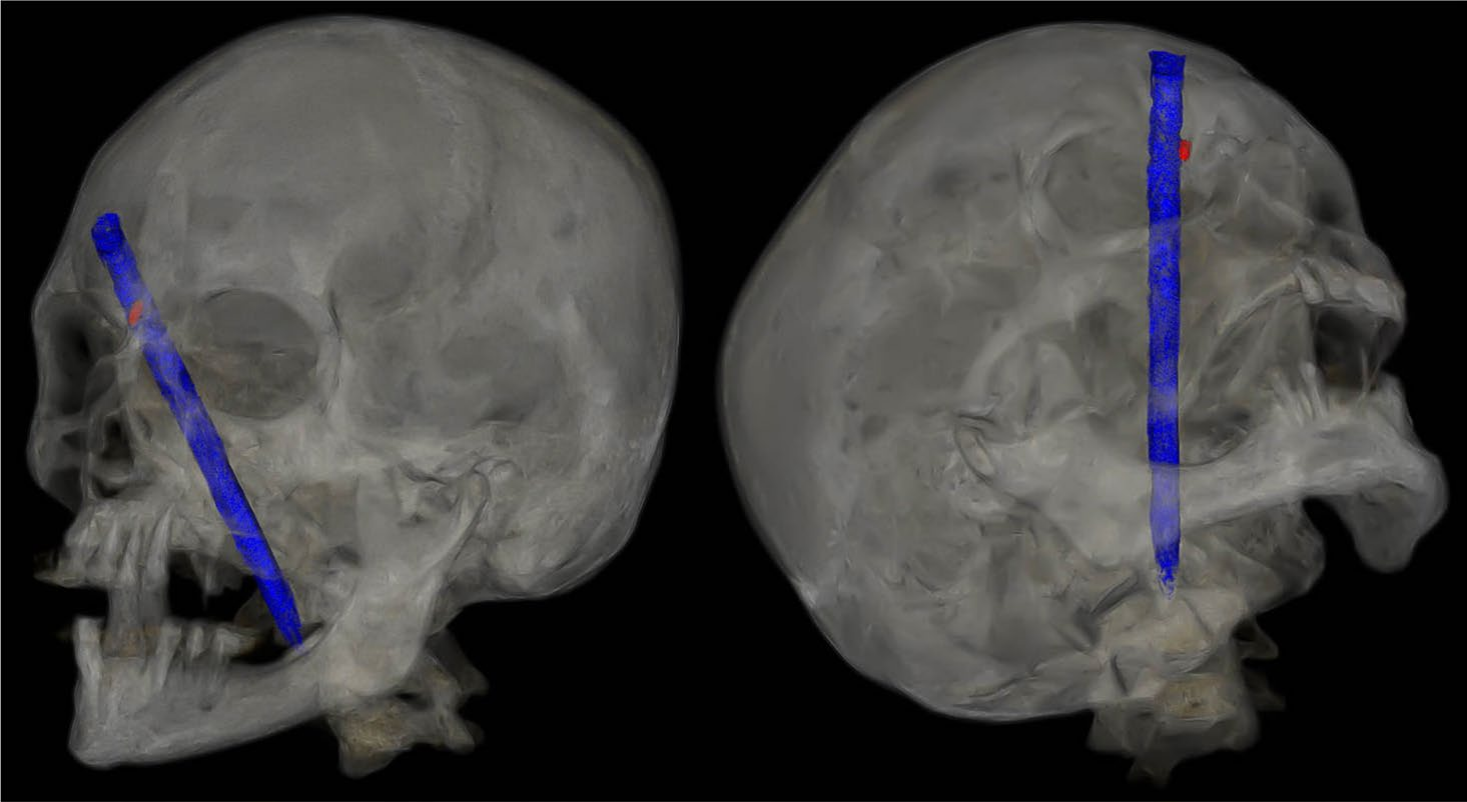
3D CT scan images showed in blue color the trajectory of the pencil from the forehead to the upper mediastinum and in red color the position of the pencil lead.
